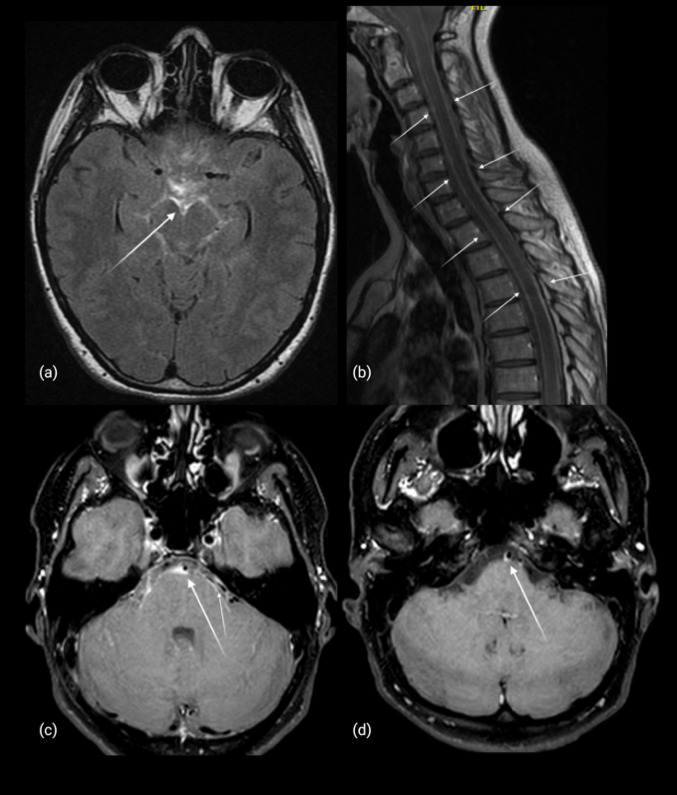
MRI of the cerebrum and neuroaxis with contrast and HRVWI-MRI sequences (2021–2022), case 1. a Post-contrast FLAIR leptomeningeal enhancement of the brainstem (white arrow), b post-contrast T1 leptomeningeal enhancement of the spinal medulla (white arrows), c initial HRVWI-MRI with concentric enhancement of the left SCA (short arrow) and BA (long arrow) wall and significant reduction of the lumen (white arrows). The left VA was occluded distal to PICA on HRVWI-MRI but had preserved flow voids on the first MRI (not shown), d HRVWI-MRI 3.5 months later with regression of the concentric enhancement around the BA and increasing lumen size (white arrow). MRI (magnetic resonance imaging), SCA (superior cerebellar artery), BA (basilar artery), VA (vertebral artery), FLAIR (fluid attenuated inversion recovery), HRVWI-MRI (MRI High resolution vessel wall imaging)
